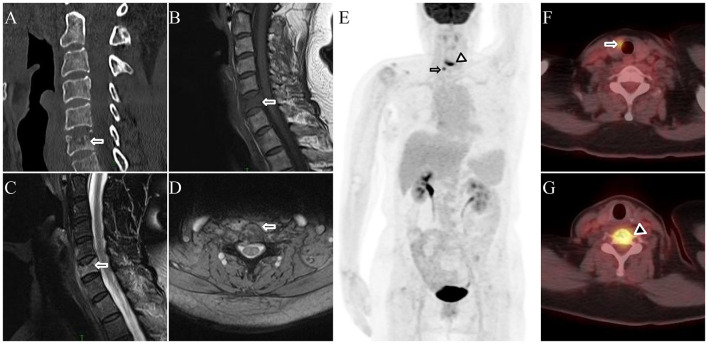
(A) CT showing a hypodense lesion with compression fracture in the C6 vertebral body. (B) T1-weighted MRI demonstrating hypointense signal in the C6 vertebra. (C) Fat-suppressed T2-weighted MRI showing hyperintense signal in the same region. (D) Axial MRI revealing surrounding soft-tissue swelling and edema. (E) Maximum-intensity projection (MIP) PET image showing 18F-FDG-avid lesions in the C6 vertebra (arrowhead) and right thyroid lobe (arrow). (F) Axial PET/CT demonstrating a hypodense lesion with ill-defined margins in the right thyroid lobe (SUVmax 6.1). (G) Axial PET/CT showing intravertebral hypodensity in the C6 vertebra with markedly increased FDG uptake (SUVmax 10.8).
